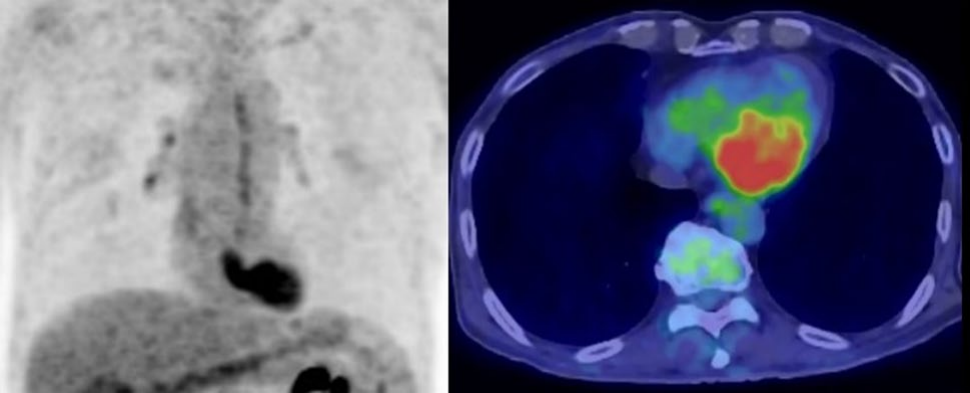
Cardiotoxicity induced by Adriamycin. Myocardial dysfunction was occurred in the patient with diffuse large B cell lymphoma treated by R-CHOP. Increased focal FDG uptake in the myocardium was confirmed during the therapy. The patient was diagnosed with cardiotoxicity induced by adriamycin
